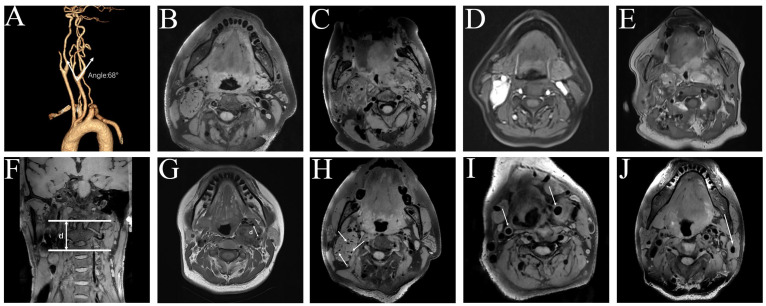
Imaging data collection. The measurement of the bifurcation angles, which was obtained in the reconstructed images of the head and neck CTA (A). The signal was relatively homogeneous on T1WI (B) and T1WI-CE (D) in soft CBTs, but heterogeneous in hard CBTs (C,E). BD was the distance from the uppermost pole of CBTs (lower straight line) to the apex of the odontoid process of the axis (upper straight line) in the coronal position sequence (F). d in subfigure (F) means BD. SD was the distance between ICA and ECA (G). d in subfigure (G) means SD. Newly formed arteries and veins are vessels in CBTs ultimately connected to the carotid artery and internal jugular vein (white arrows) (H). PFS was the complete fat structure of the outermost layer of the artery (I). The PFS of the right carotid artery was intact, but the PFS of the left carotid artery was eroded by CBTs. The EJV (white arrow) on the left side was dilated (J).
